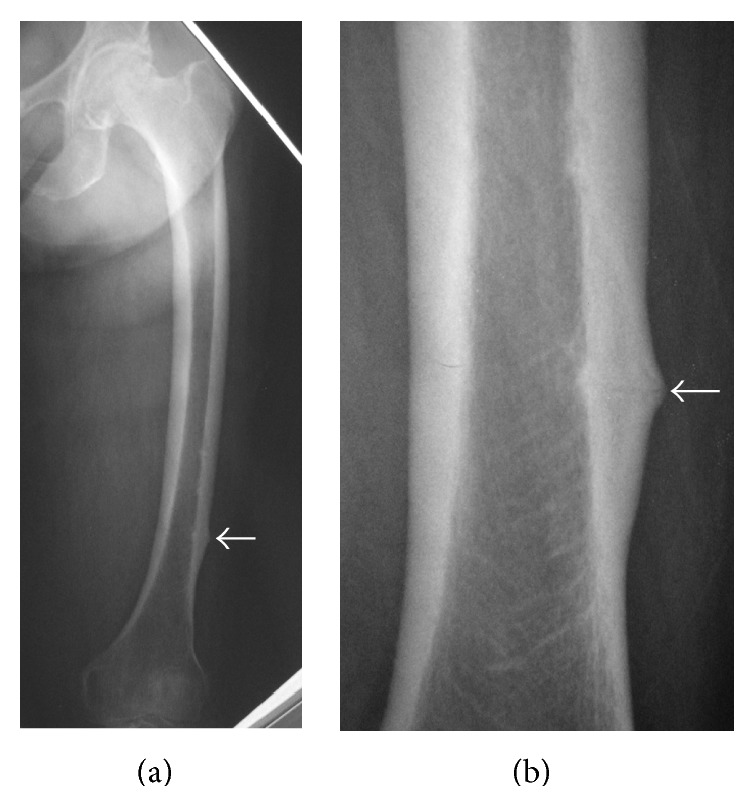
Anteroposterior radiographs of the left femur. (b) is a magnified view of (a). They showed some thickening of the femoral cortex and a horizontal line resembling a fracture over the outer cortical bone of the distal diaphysis.
